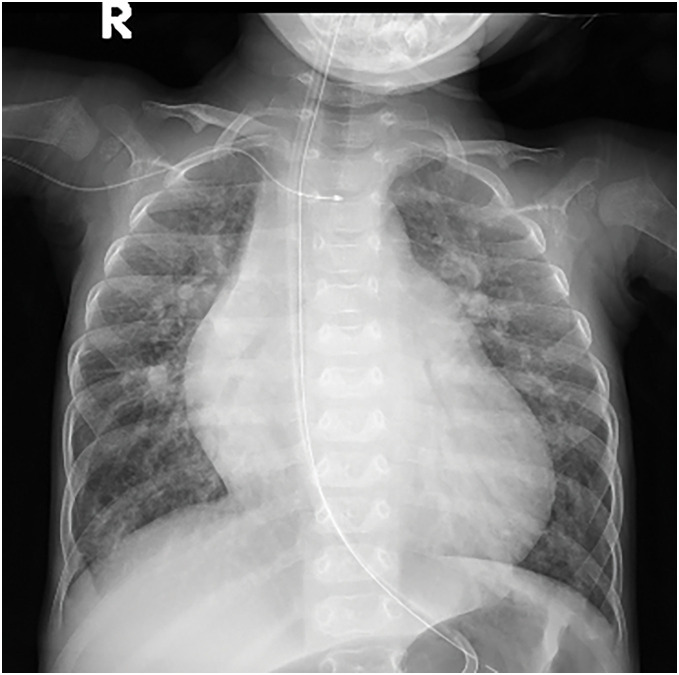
Preoperative chest radiograph of patient 2. There is significant cardiomegaly and pulmonary congestion.
